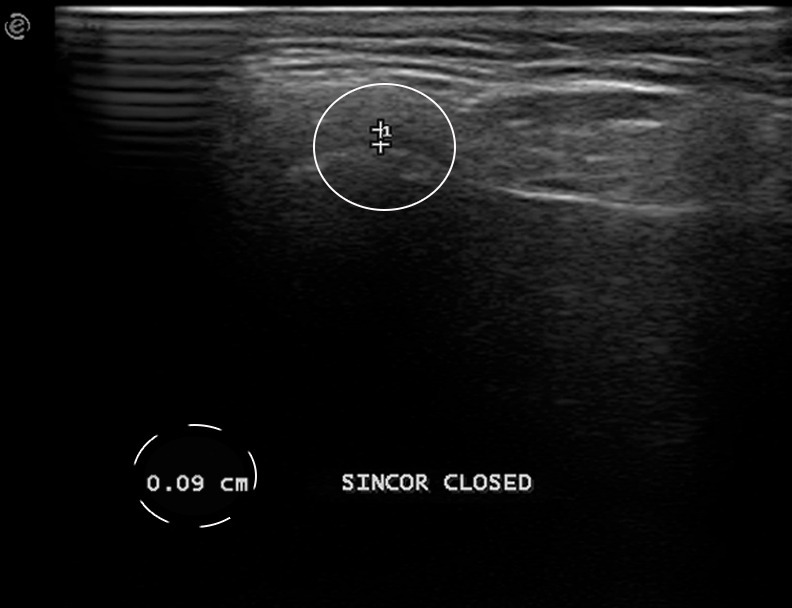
Ultrasound image of a left (SIN) temporo-mandibular joint. A linear probe in Coronal (COR) direction was used and the patient was in a closed-mouth position. The LPAS, which is the hypoechoic strip measured in orthogonal mode over the most lateral point on the condyle cortical, is identified between the two reference points (+). The width of the LPAS is 0,09 cm as showed in the dashed circle. LPAS, lateral periarticular space.
